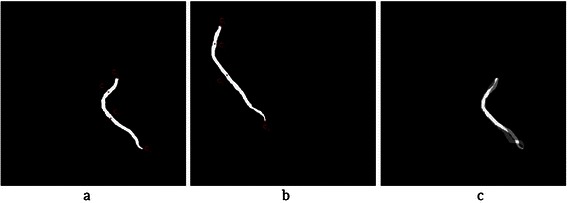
The result of the proposed method for main coronary artery registration with control points. (a) Main coronary artery segmented and labeled from CTA including control points. (b) Main coronary artery segmented and labeled from the angiogram including control points. (c) The result of applying the proposed method.
